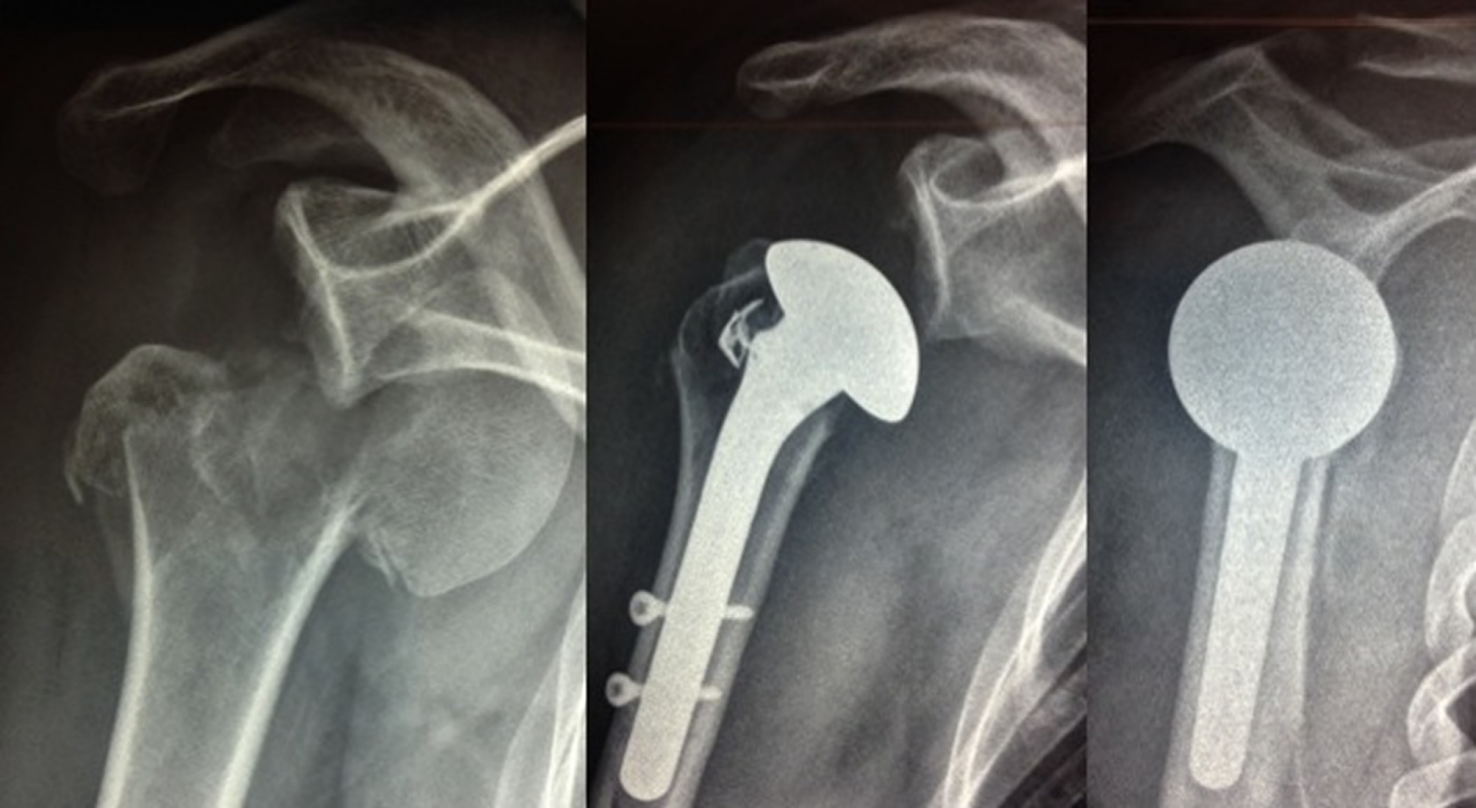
Four part dislocated fracture in a 55-year-old patient (right shoulder) and postoperative X-ray.
